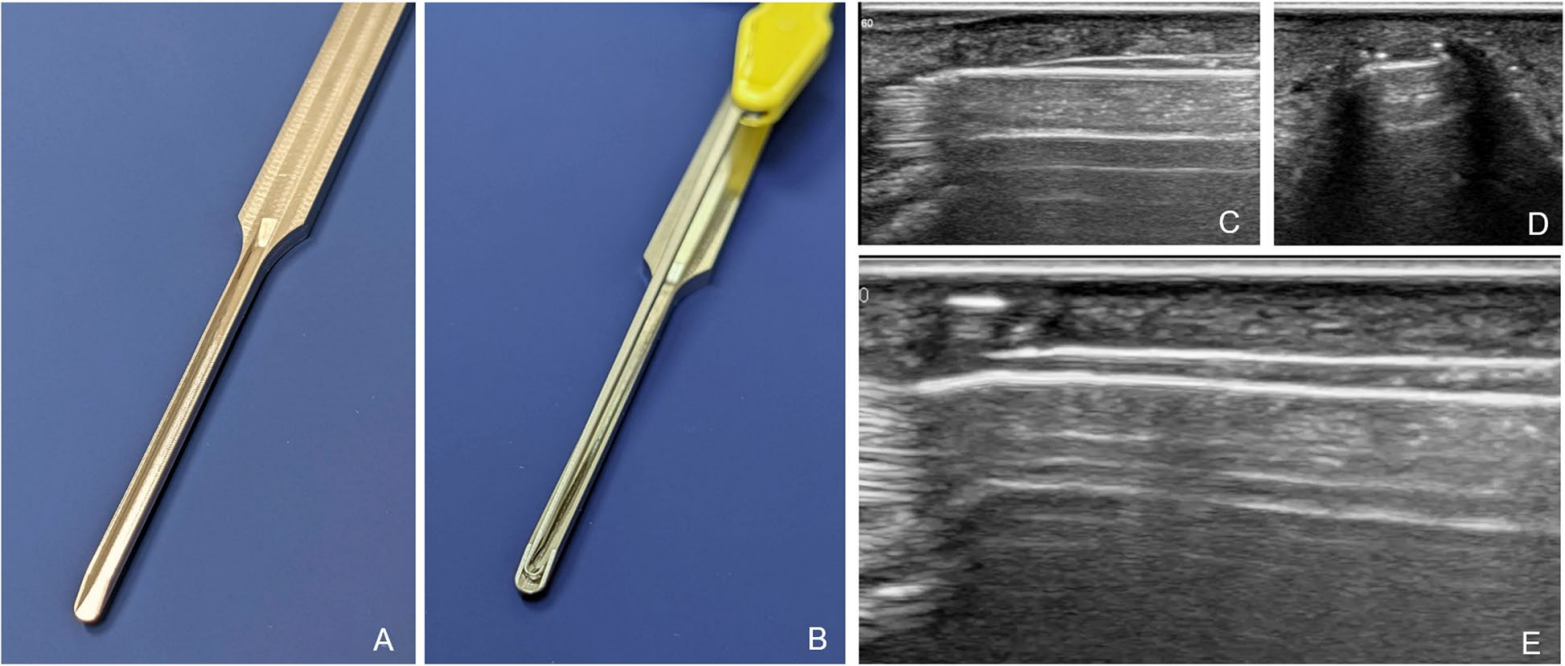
A-E Guide instrument and hook knife with sonographic view. A Guide instrument, as of November 2019; B guide instrument shown with inserted hook knife; C longitudinal and D axial sonographic view of guide instrument in the intrasheath position; E hook knife placed upright within channel just proximal to the A1 pulley
